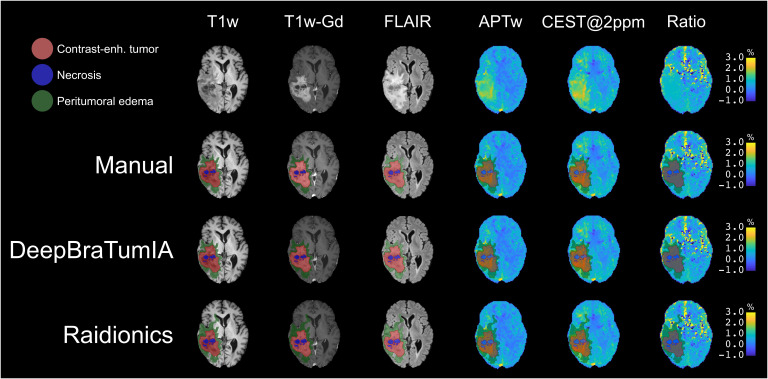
A single slice of the whole tumor segmentations performed in a 61-year-old patient with glioblastoma, non-methylated MGMT promoter. Images include conventional T1-weighted MRI before (T1w) and after (T1w-Gd) contrast administration, fluid-attenuated inversion recovery (FLAIR), fluid-suppressed amide proton transfer weighted map at 3.5 ppm (APTw), fluid-suppressed CEST signal map at 2.0 ppm (CEST@2ppm) and the APTw/CEST@2ppm ratio. The contrast-enhancing tumor (red), necrosis (blue) and peritumoral edema (green) are segmented manually and automatically with DeepBraTumIA and Raidionics.
